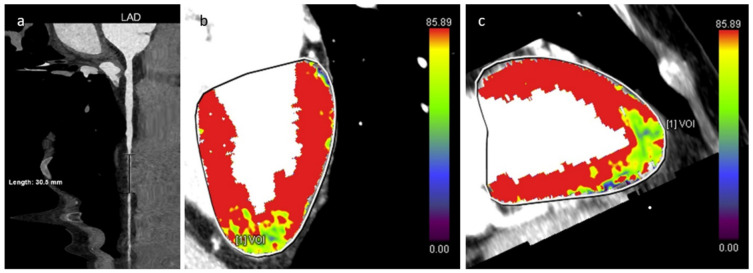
A superficial myocardial bridge which is 3 cm long (a) and cause apical hypoperfusion at stress dynamic computed tomography myocardial perfusion (b,c).
